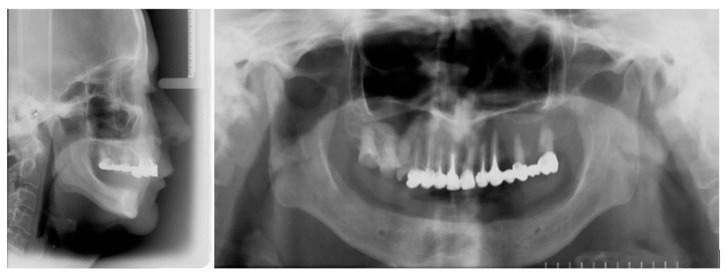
Initial radiological assessment was performed using both lateral cephalometric and panoramic radiographs.
